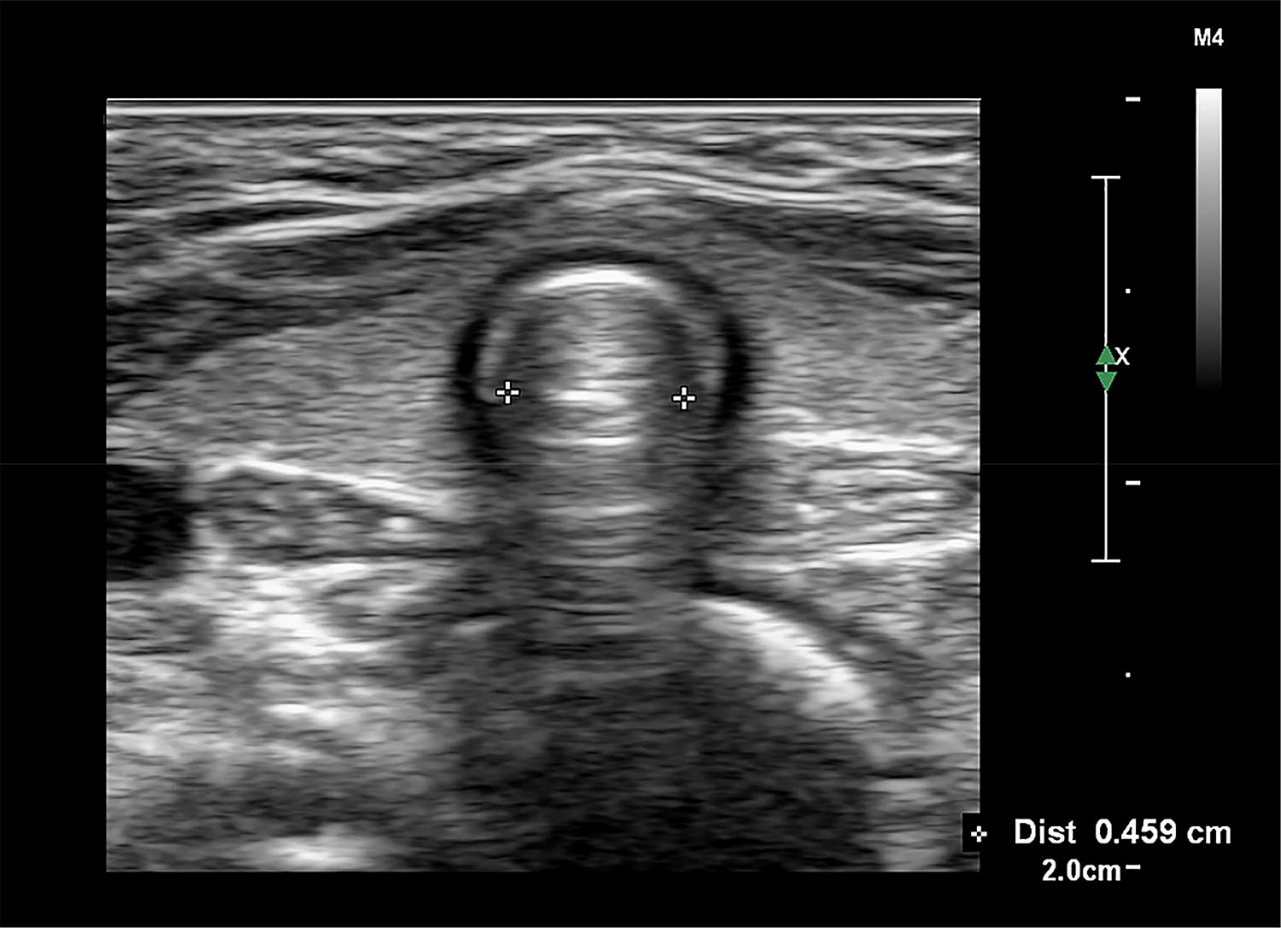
Ultrasonographic measurement of the transverse subglottic diameter of the cricoid cartilage. The cricoid cartilage appears as a round hypoechoic structure with hyperechoic edges, composed of perichondrium. The transverse subglottic diameter is determined by measuring the dimension of the air-mucosa interface (between two crosses) within the bilateral inner margin of the cricoid cartilage.
